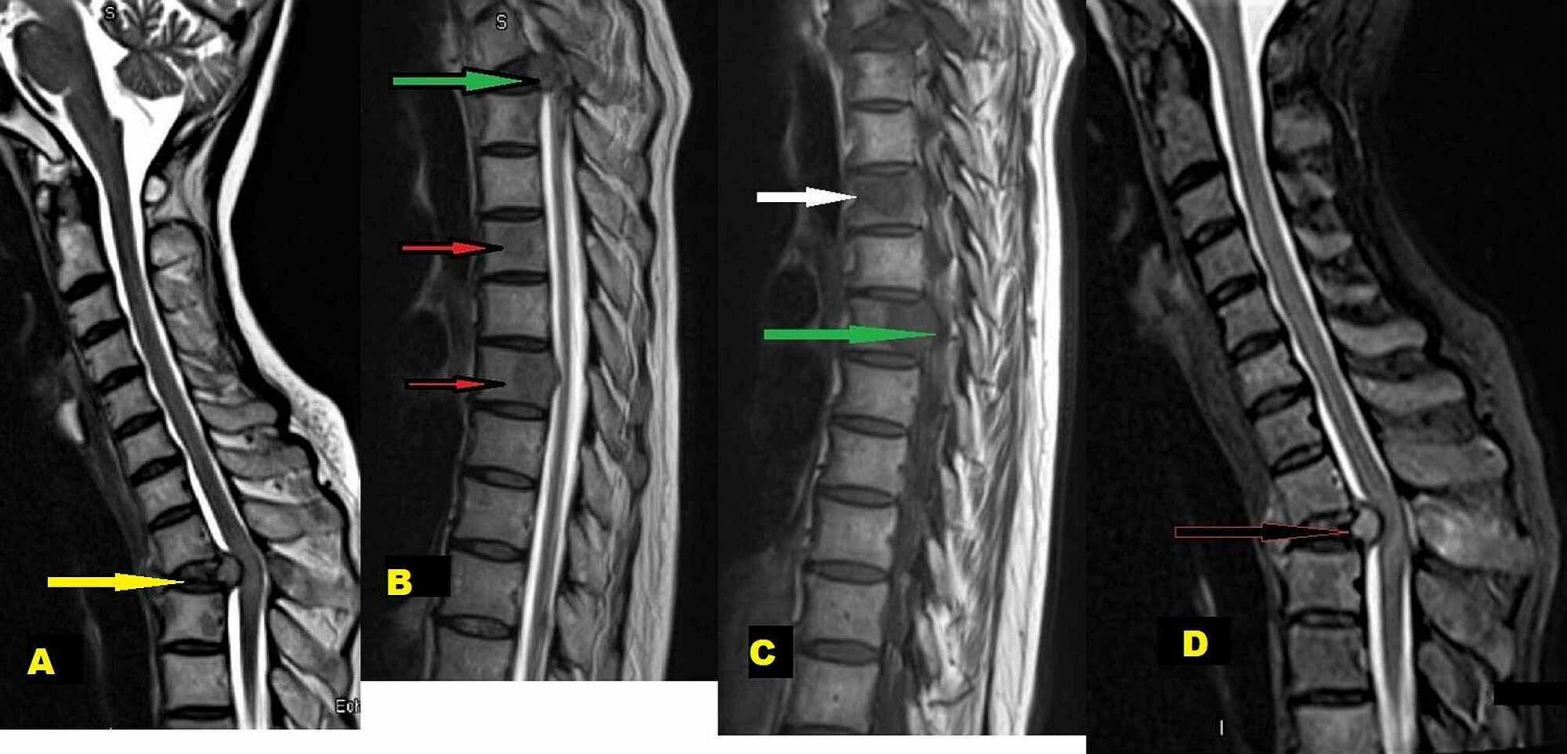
MRI Spine A) (Para-sagittal view) T2-weighted images showing compression at T1-T2 spinal level (yellow arrow) B) Multiple spinal bony metastasis (red arrows) with compression on the anterior spinal cord (green arrow) C) T1-weighted MRI images showing body metastatic (white arrow) and pedicles metastatic involvement (green arrow) D) Extradural compression on the cord pushed posteriorly (arrow)
